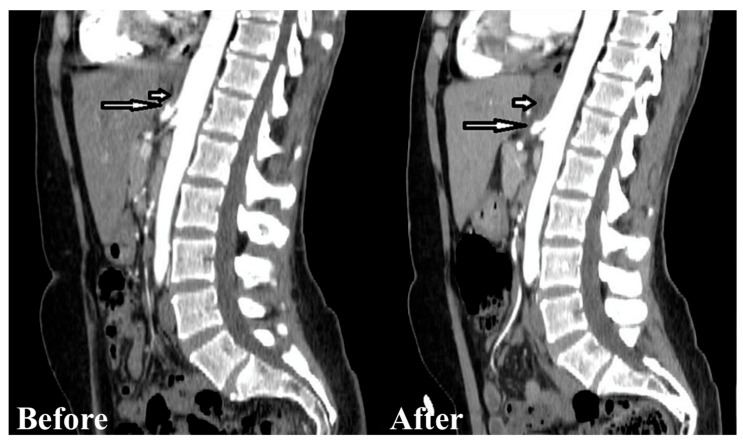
Before-surgery sagittal reconstruction of contrast-enhanced computed tomography demonstrates a short-segment stenosis of the celiac trunk with a characteristic hooked configuration and post-stenotic dilatation (long arrow). Thickening of the median arcuate ligament is also evident (short arrow). After-surgery sagittal computed tomography shows complete release of the celiac trunk stenosis (long arrow). Postoperative soft-tissue edema is visible in the surrounding tissues (short arrow).
